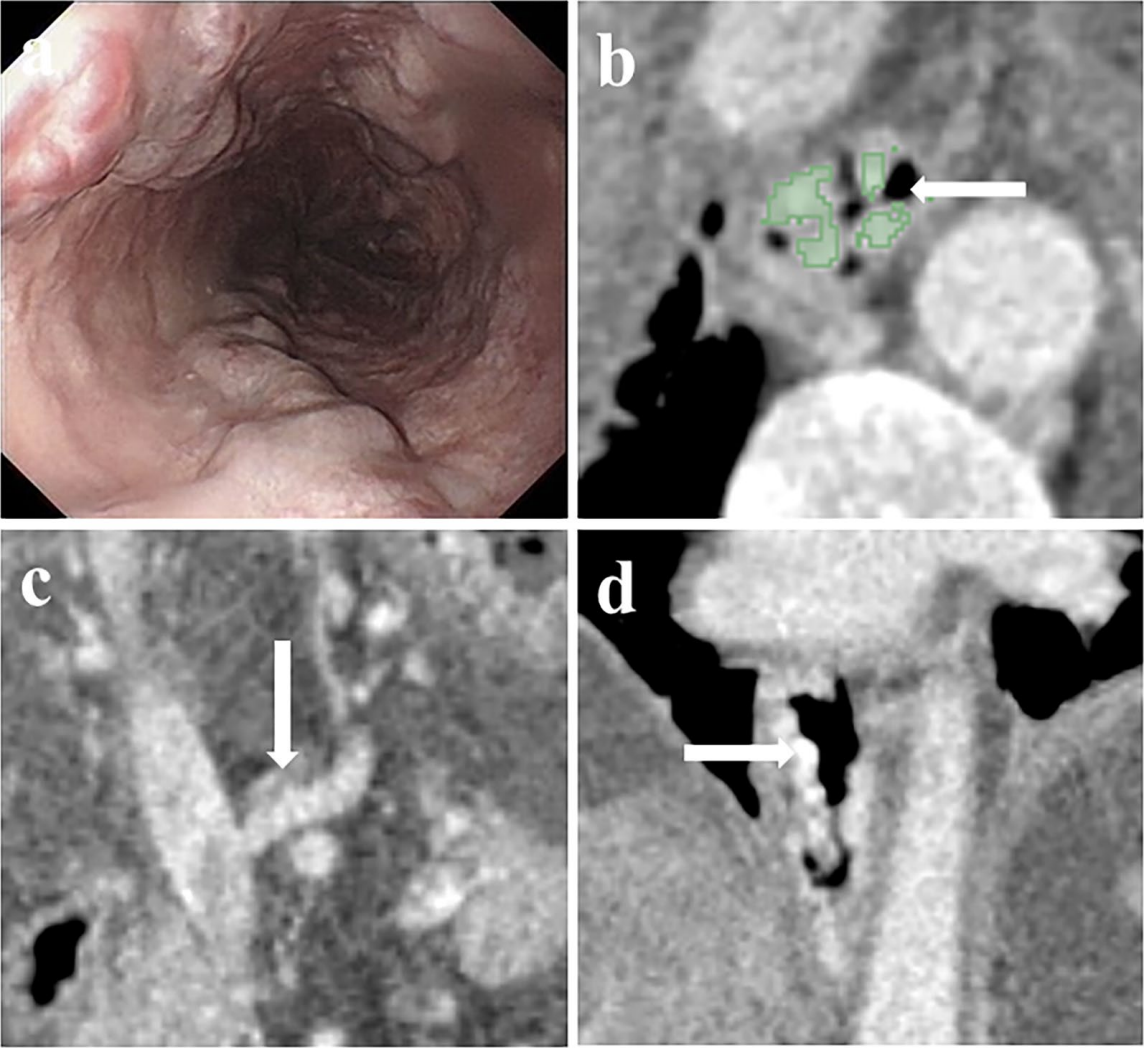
Severe EV according to endoscopy in a 68-year-old man with liver cirrhosis (severe of endoscopy, maximum minor axis 6.28 mm). a The endoscopic image shows severe nodular varices; b the cross-sectional surface area (CSA) of EV (dyeing area) in the transverse section is depicted in 3D slicer; c the axial CT image shows the left gastric vein (LGV) originating from the portal vein (arrow); d 3D reconstruction has a satisfactory performance in visualizing severe EV of the lower esophagus (arrow). EV, esophageal varices
